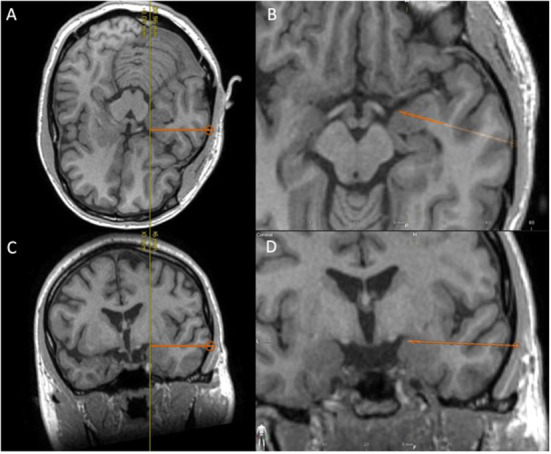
Using T1-weighted MRI, we demonstrate the proposed placement of a temporal electrode that would end in the temporal piriform cortex; (A) Peri-axial in-line view, (B) enlarged peri-axial view, (C) Peri-coronal in-line view, and (D) enlarged peri-coronal view.
